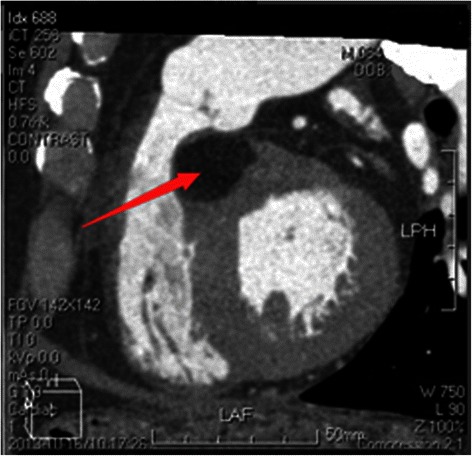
Cardiac CT images with contrast confirmed the existence of a round-like hypodense mass (−117Hu), measuring 37 × 21 mm in its largest diameter, superior to the IVS and anterior to the right coronary sinus of aorta.
